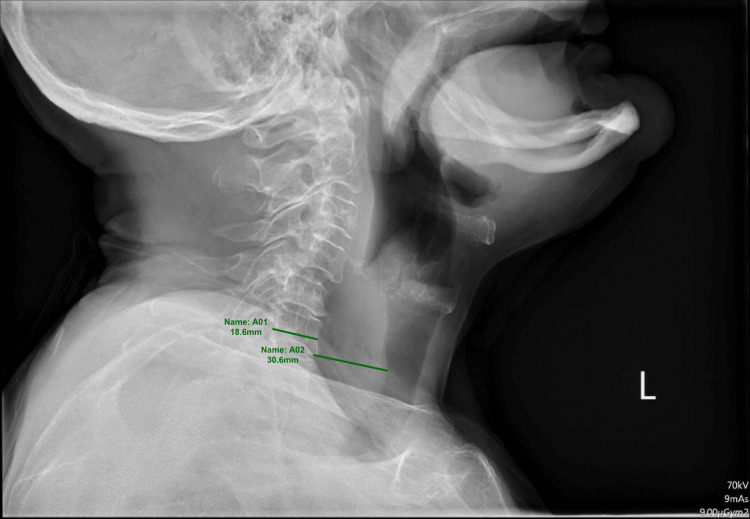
X-ray soft tissue neck A01: C6 mid-vertebral anteroposterior diameter (18.6mm)
